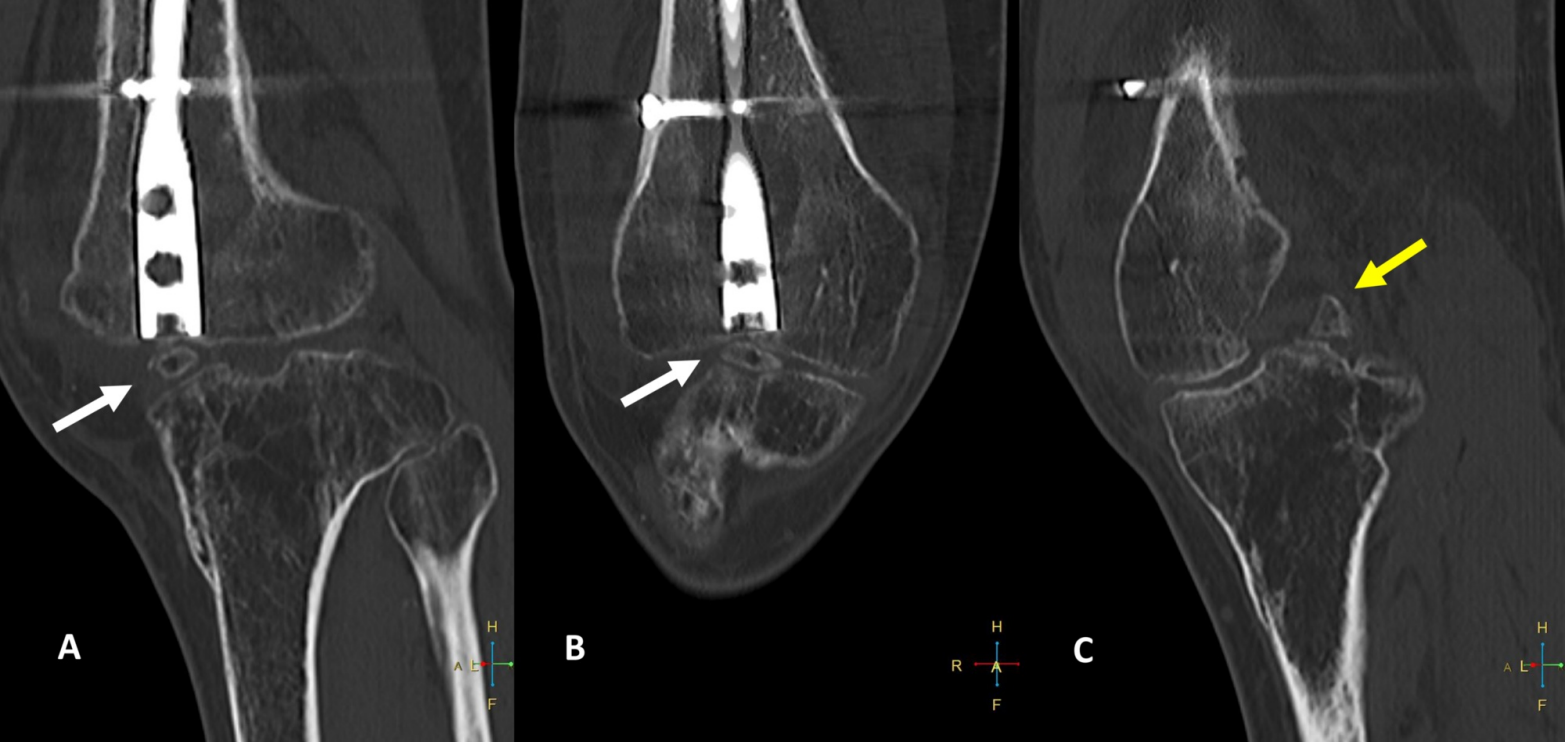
Two-dimensional computed tomography images of the knee A: coronal image showing heterotopic bone inferior to the lower end of the nail. B: sagittal section showing the distal nail and heterotopic bone in relation to the end of the nail. C: shows nonunion of avulsion fracture of PCL. White arrow - heterotopic bone; yellow arrow - PCL avulsion; PCL - posterior cruciate ligament
